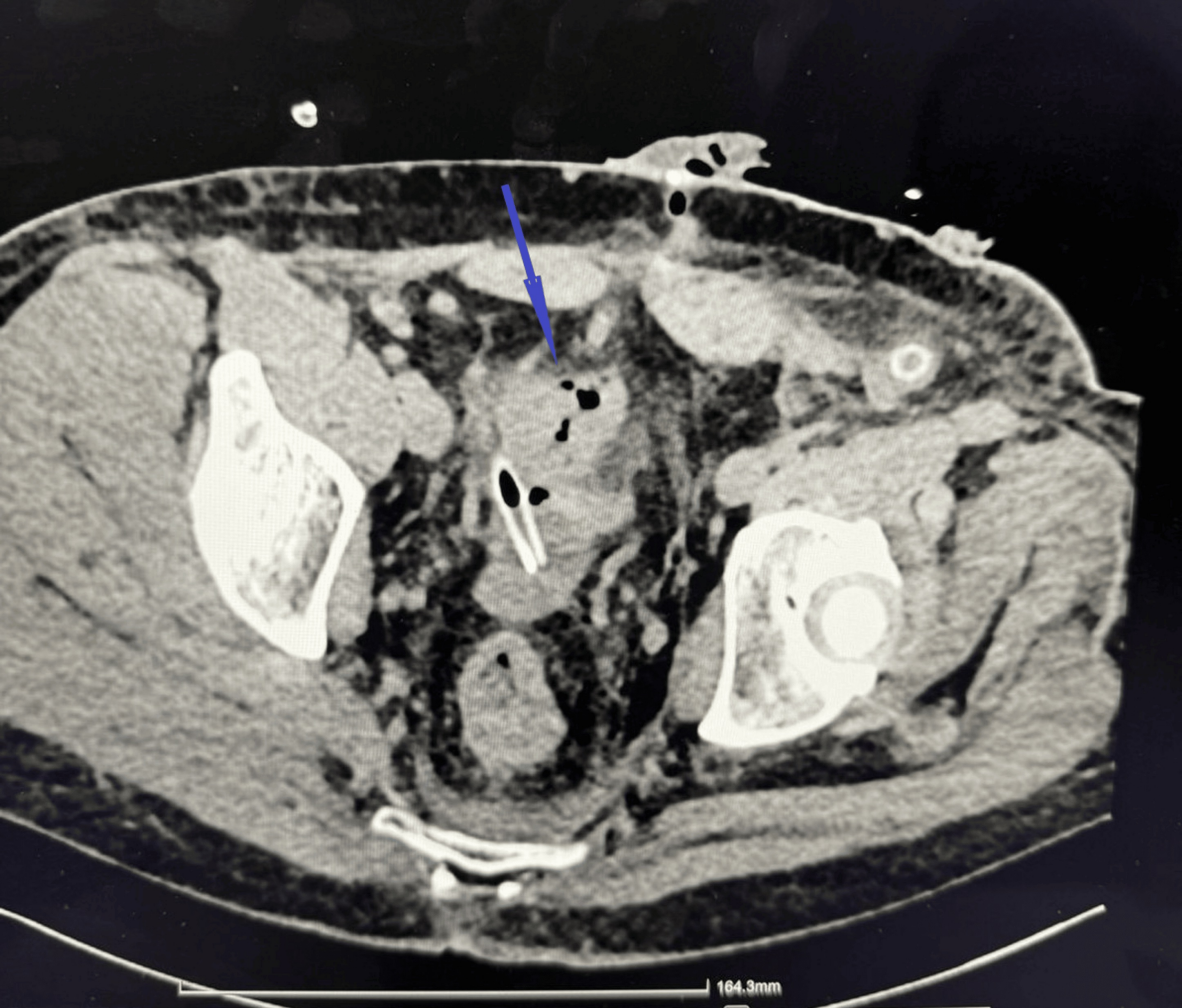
Abdominal CT Abdominal CT demonstrating a wall defect at the left sigmoid colon stump (blue arrow), measuring up to 4 mm in transverse diameter, with an adjacent isolated gas-density focus. A small volume of free fluid is present in the pelvic cavity near the superior margin of the urinary bladder, with associated gas-density foci, concerning for an anastomotic leak.
